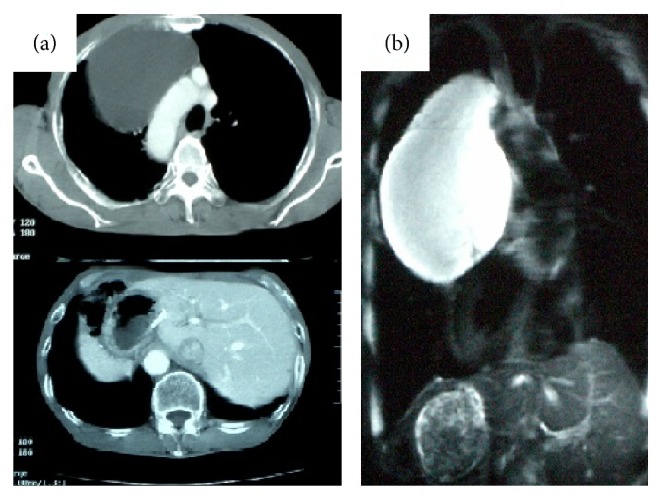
(a) Enhanced computed tomography scan of the whole body shows a right mediastinal cystic mass with a right-sided aortic arch and liver, consistent with situs inversus totalis. (b) T2-weighted magnetic resonance imaging scan of the chest shows a homogenous, high-intensity area adjacent to the right side of the mediastinum.
